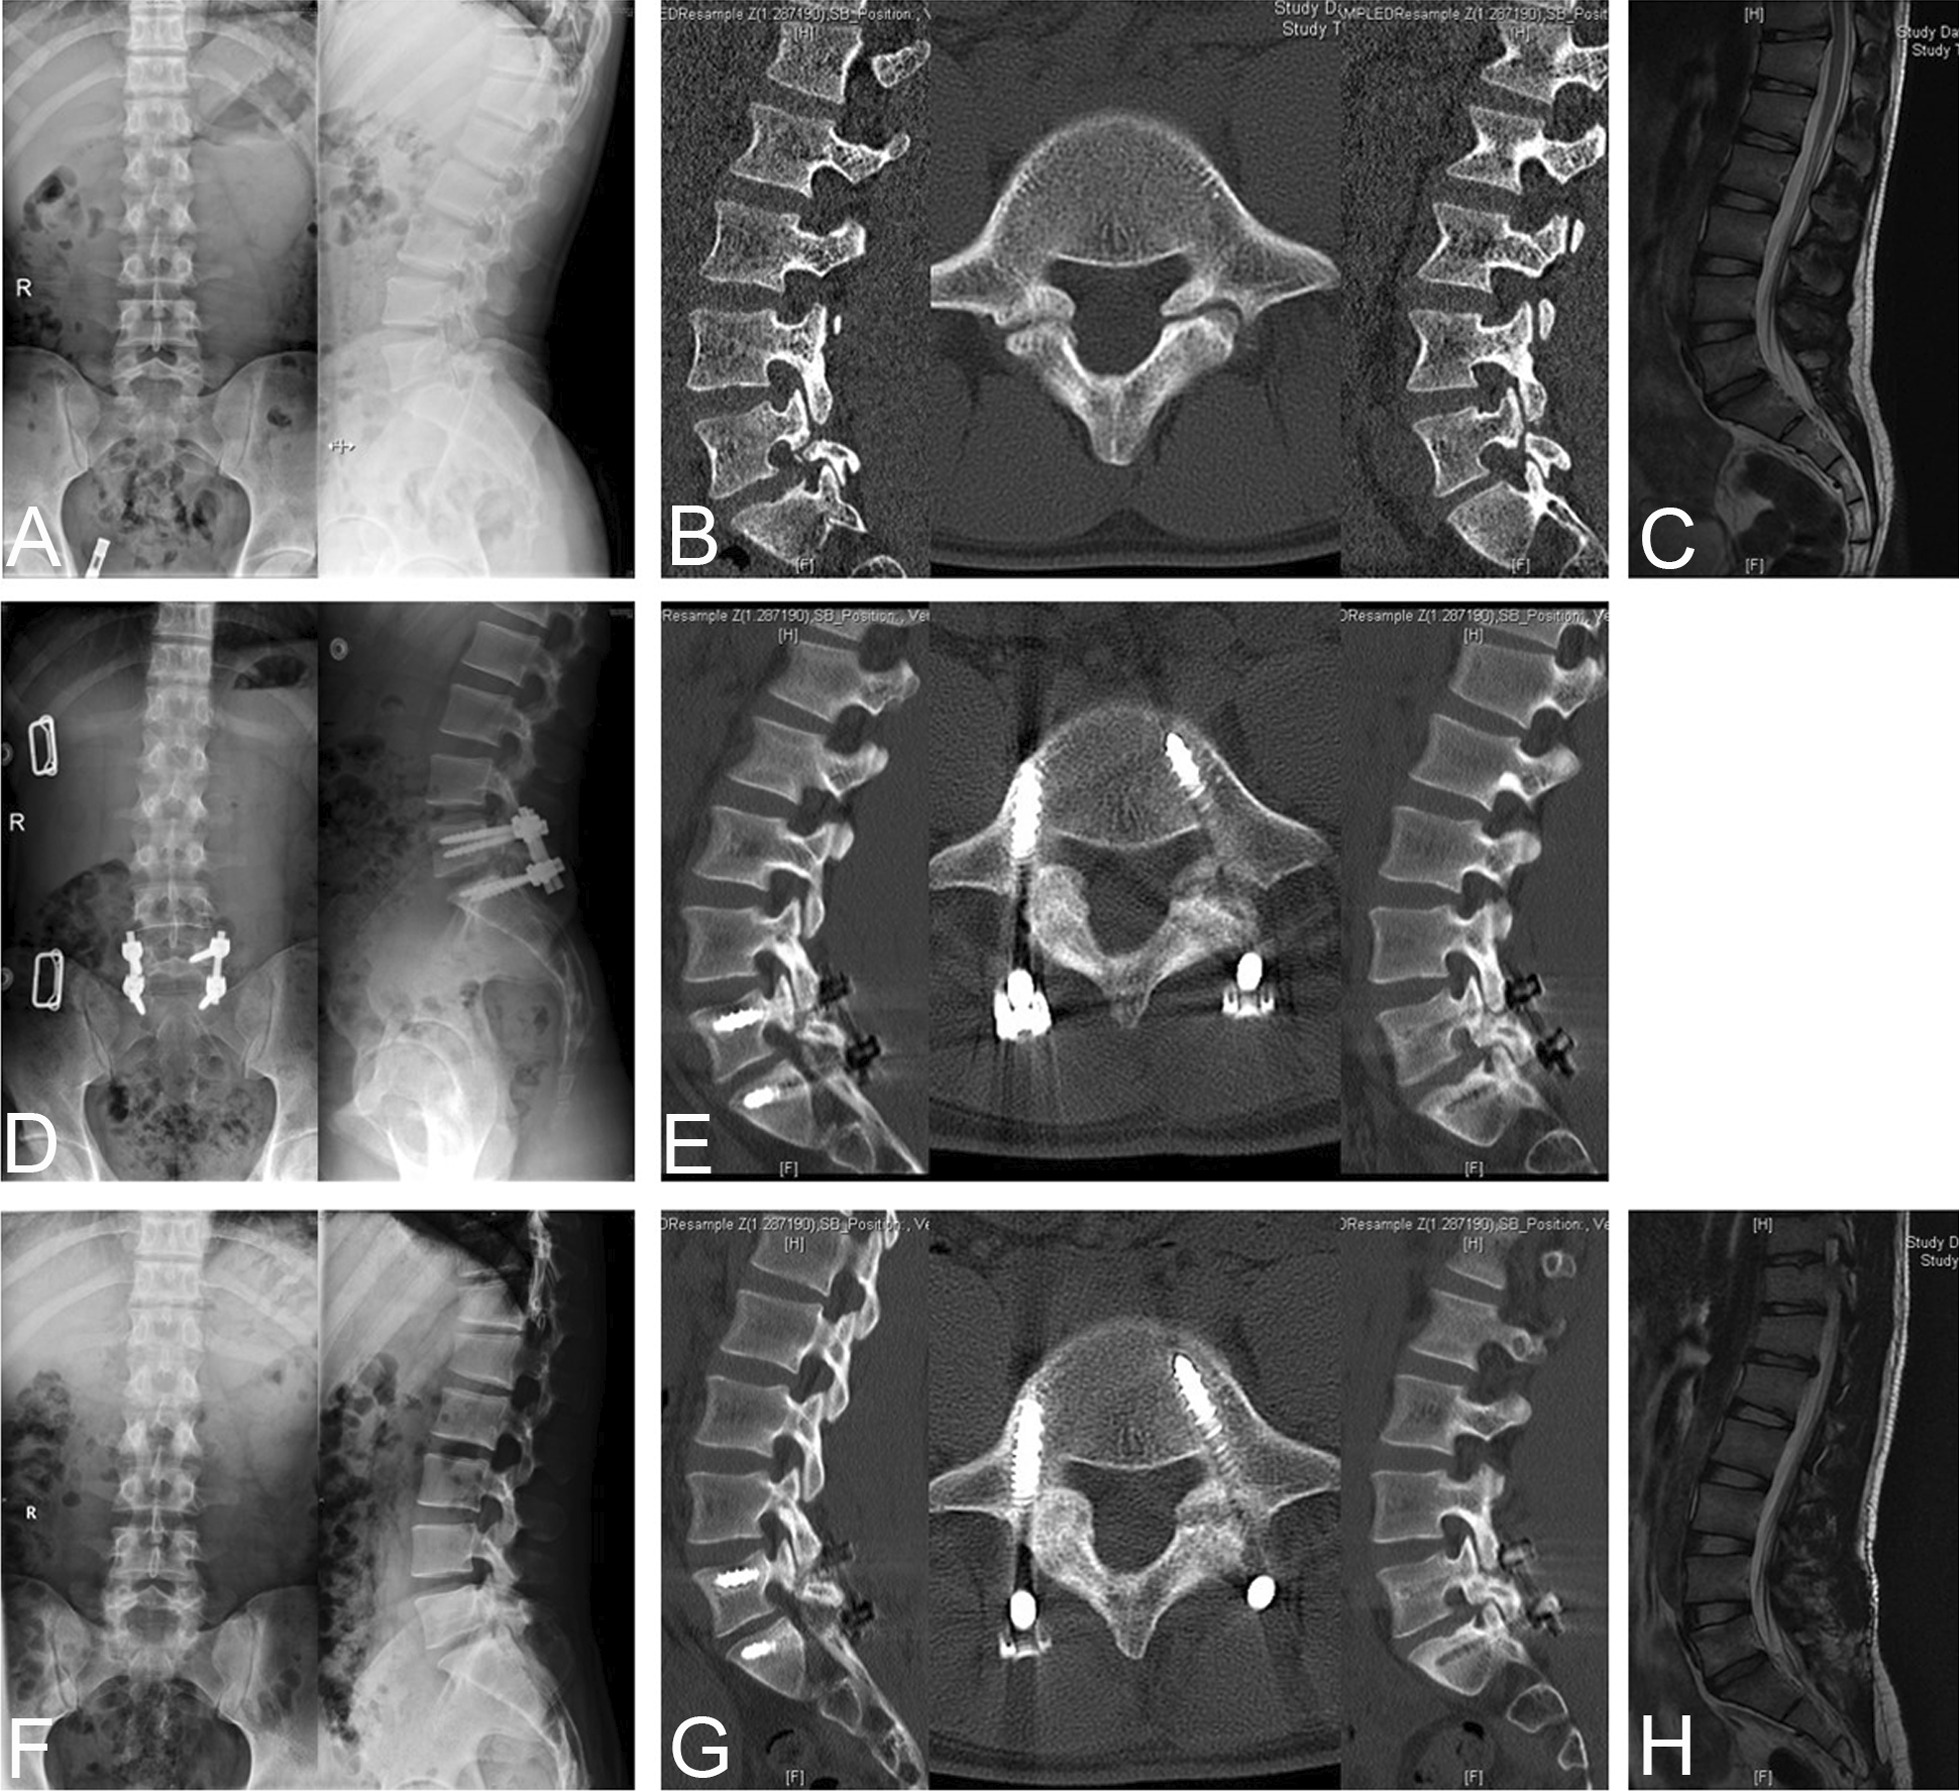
Preoperative and postoperative images obtained in a 19-year-old man with L5 spondylolysis. a–c Preoperative radiographs, CT scan and MRI. d One-week postoperative radiographs. e 9-Month follow-up CT scan. f 15-Month radiographs showed that the lumbar instrumentations had been removed. g–h 15-Month postoperative CT scan and MRI
